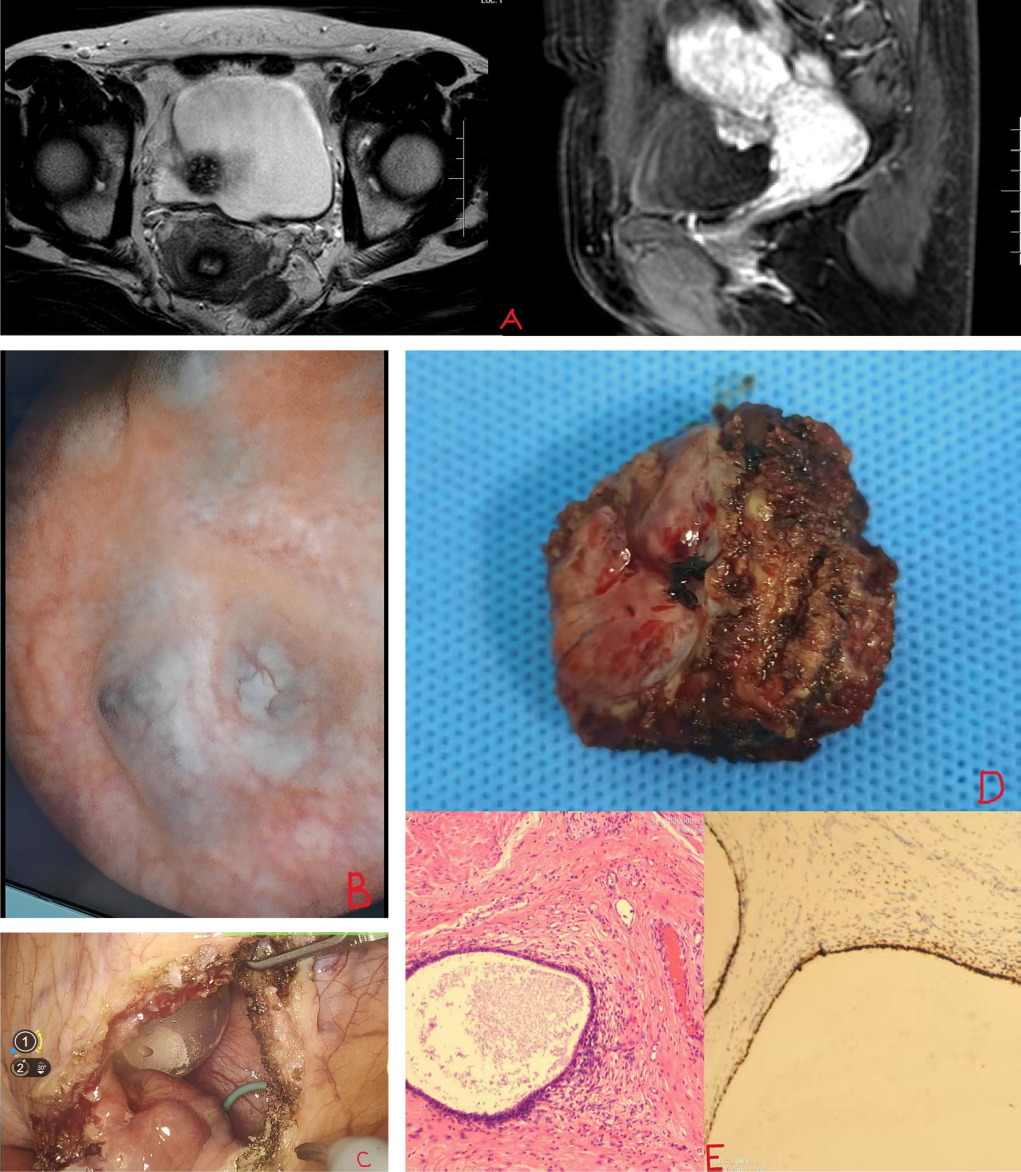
(A) MRI shows bladder mass. (B,C) Blue-purple nodules at the base of the bladder. (D,E) Bladder endometriosis and PR(+).
